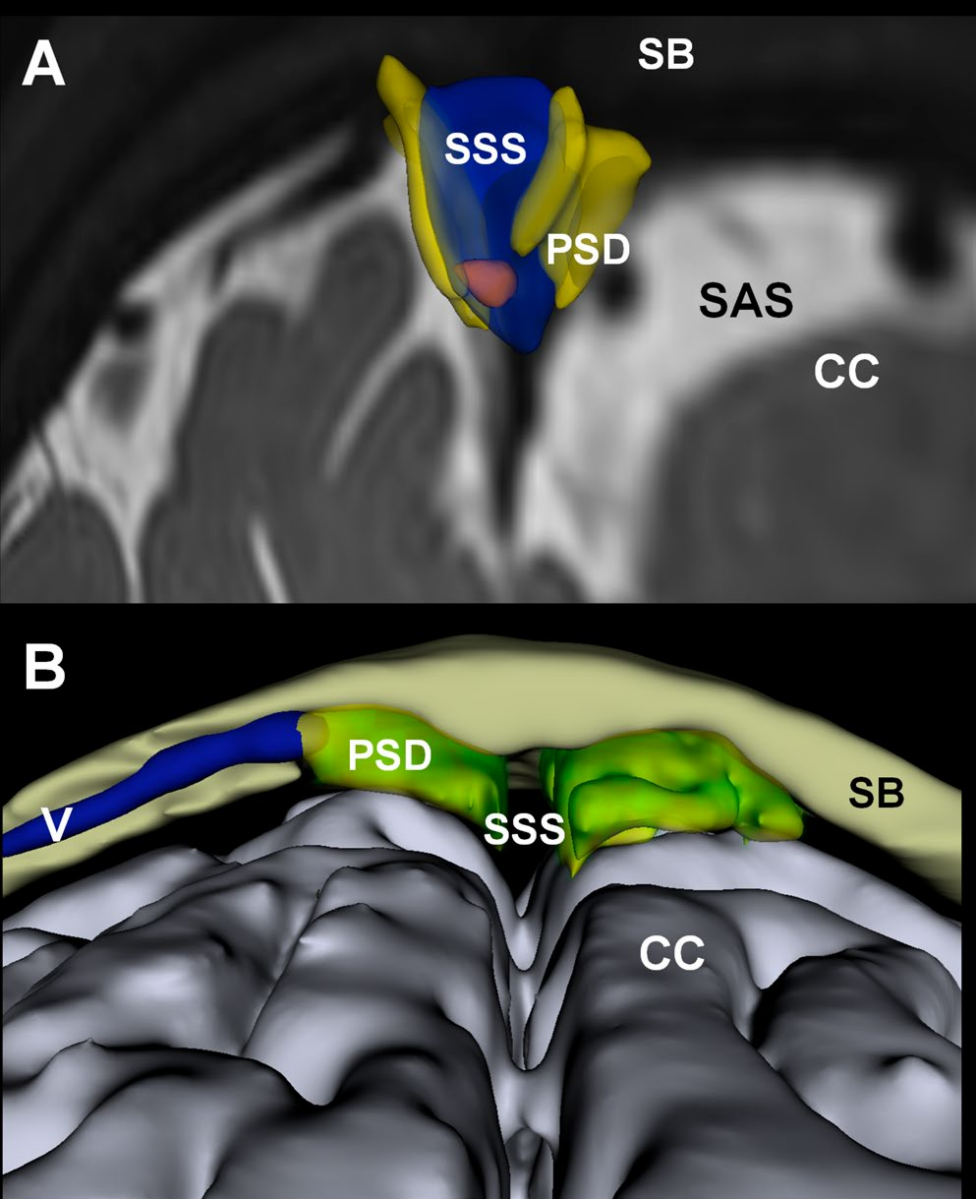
The parasagittal dura. An MRI contrast agent utilized as a cerebrospinal fluid (CSF) tracer enriches the CSF within the subarachnoid space (SAS) and traverses the arachnoid membrane into the dura mater along the superior sagittal sinus (SSS), known as the parasagittal dura (PSD). This phenomenon is depicted here through 3D images generated from T2-FLAIR images, co-registered with brain segmentation and CSF tracer enhancement from T1 GRE at 48 h post-intrathecal tracer injection. (A) The superior sagittal sinus (SSS) is highlighted in blue, while the parasagittal dura (PSD) is depicted in yellow. (B) The PSD may extend into the marrow of the skull bone (SB). Additionally, a vein (V) within the diploic space is shown. CC: Cerebral cortex. Images by Tomas Sakinis, MD, Department of Radiology, Oslo University Hospital-Rikshospitalet.
